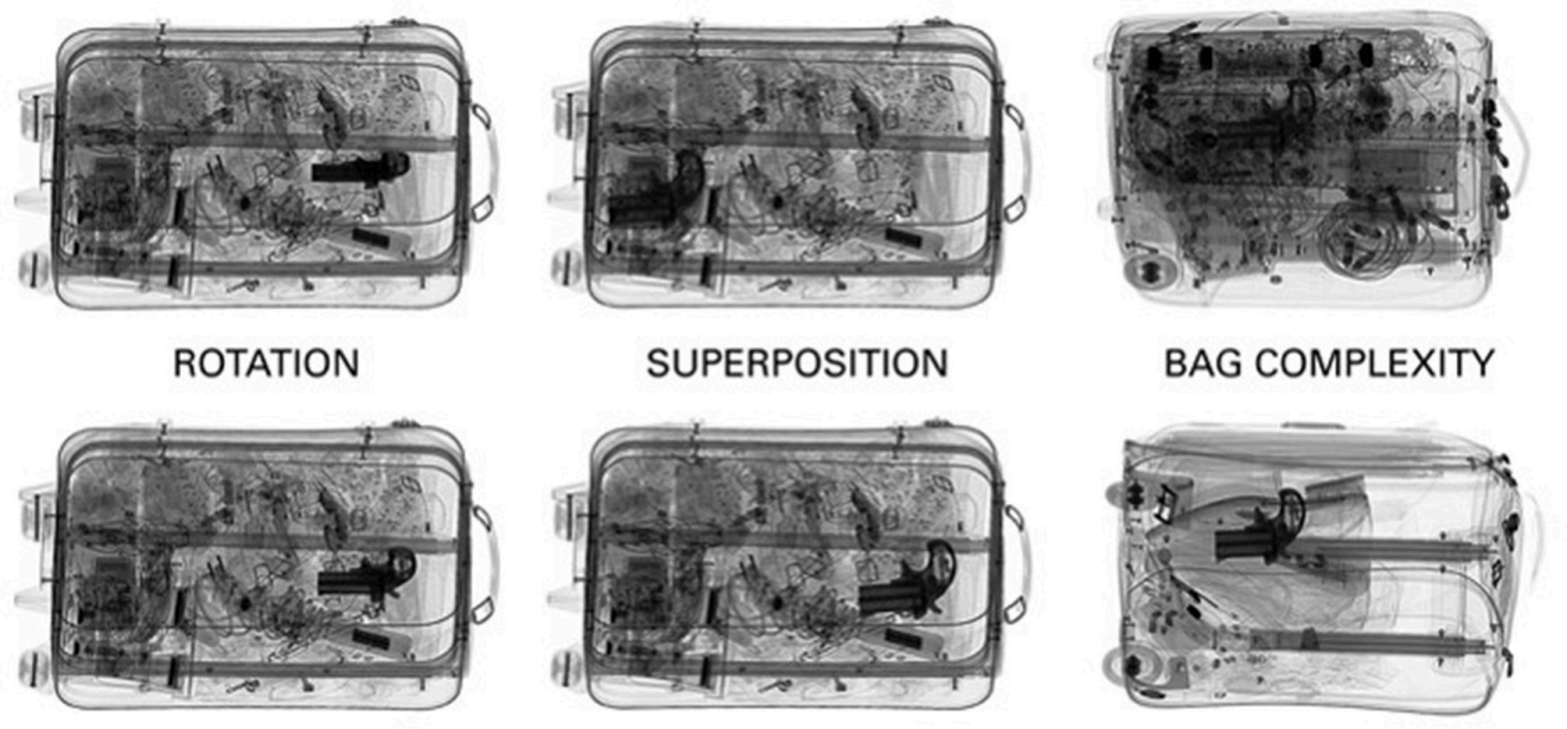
Examples of X-ray images varying in rotation of the threat object (viewpoint effect), superposition, and bag complexity.
